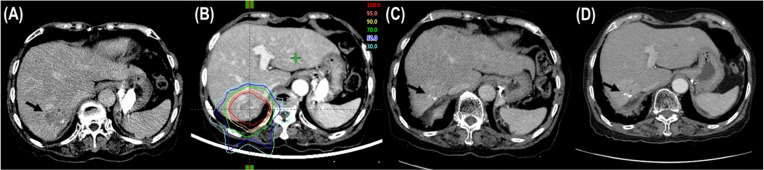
Tumor response after proton beam therapy (PBT). (A) CT scans prior to PBT showing the tumor (arrow). (B) The patient was treated with PBT. (C) CT scans at 6 months after PBT showing shrinkage of the tumor (arrow). (D) CT scans at 12 months after PBT showing complete response of the tumor (arrow).
